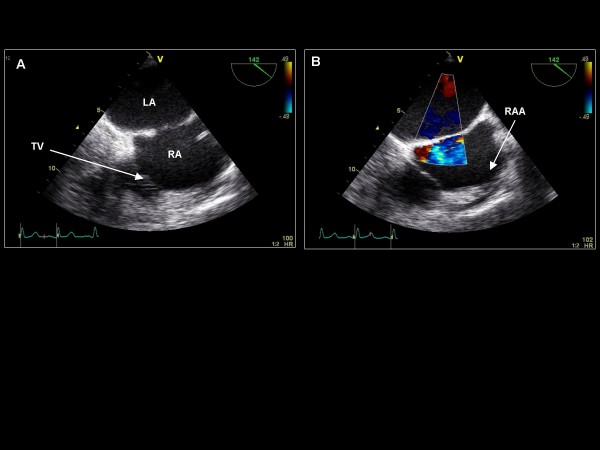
Imaging plane 135°, (A) The left atrium (LA), interatrial septum, right atrium (RA), and tricuspid valve (TV) are seen (B) The right atrial appendage (RAA) is identified and color Doppler is applied over the interatrial septum demonstrating no interatrial flow.
